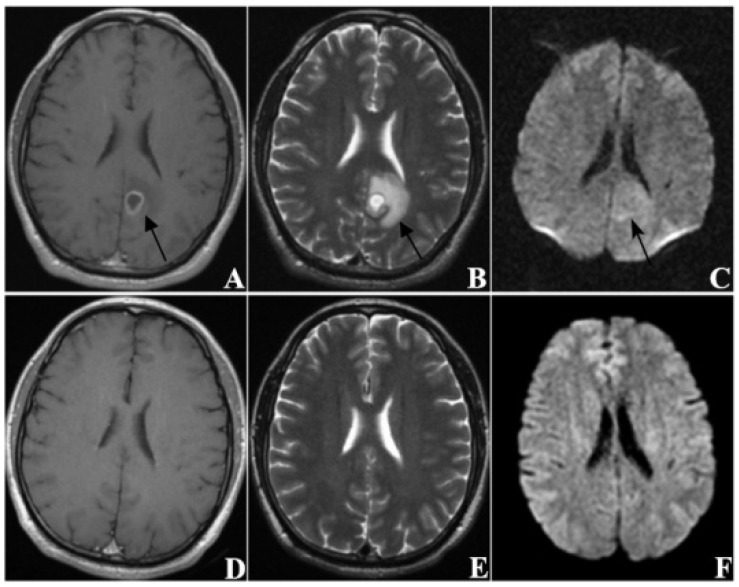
A sixty-three-year-old male patient (KPS = 80) with brain metastases (*n* = 1) from lung cancer. The primary tumor had been completely removed without recurrence and no extracranial metastasis was found. (A–C) are MRI images taken before SRS treatment, and (D–F) are MRI images taken at follow-up 63 days after treatment ((A,D) from CE-T1WI; (B,E) from T2WI; (C,F) from DWI). The tumor was located in the left occipital lobe and showed ring-like enhancement. The EI was 3.55. After treatment, the tumor disappeared, thus this patient was classified as CR.
